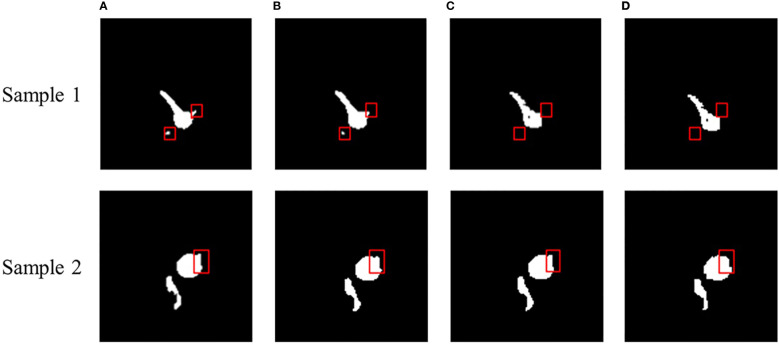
Sample segmentation effect of MRI images. (A) Real label, (B) MRSwinUNet, (C) TransUNet-D, (D) SwinUNet-D.
